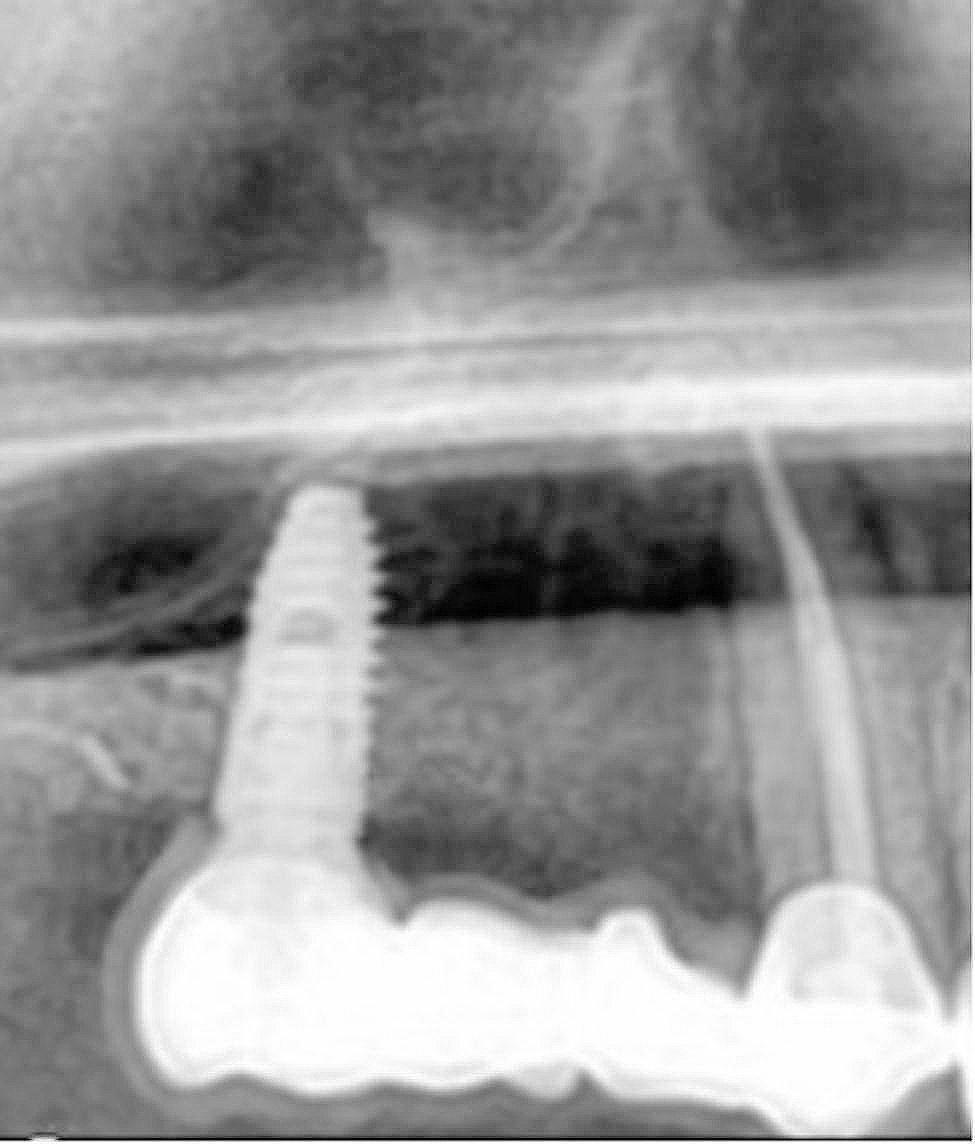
(A) Radiograph at baseline, (B) Radiograph at 12 months showing 1mm bone loss, (C) Radiograph at 24 months showing 1mm bone loss
